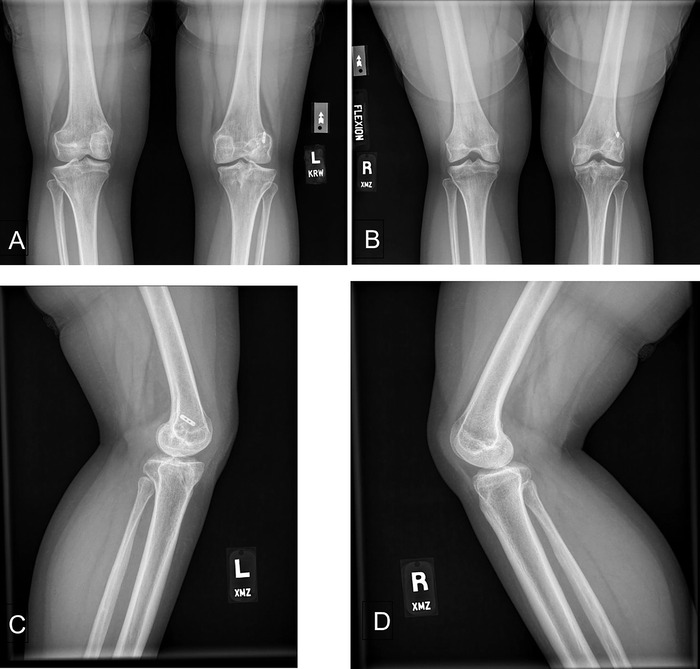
Preoperative anteroposterior radiographs of both knees in (A) standing view and (B) at 45° flexion show prior anterior cruciate ligament reconstruction tunnels along with Endobutton fixation device (Smith & Nephew plc) over the lateral distal femur. Lateral radiographs of the (C) left and (D) right knees show patellar aplasia.
